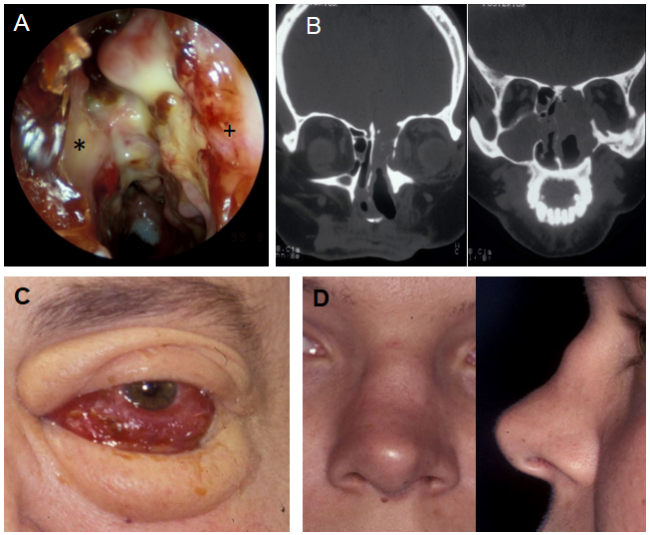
A) Nasal cavity in GPA with granulations, destruction, bacterial infection, left nasal cavity (choanal #, nasal septum*, lateral nasal wall+), B) CT scan of the same patient, destruction of the orbital wall and frontal skull base, C) The same patient, clinical aspect, D) Saddle nose deformity in GPA
